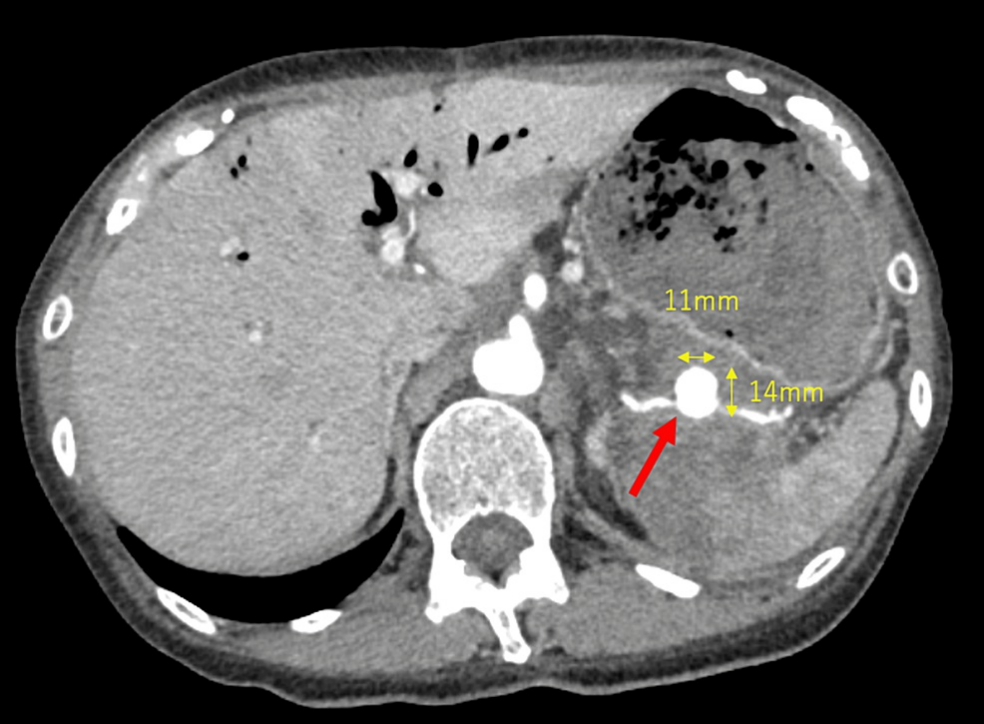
CT findings upon arrival A 14 mm x 11 mm aneurysm with surrounding hematoma in the proximal third of the splenic artery (red arrow) CT: computed tomography
